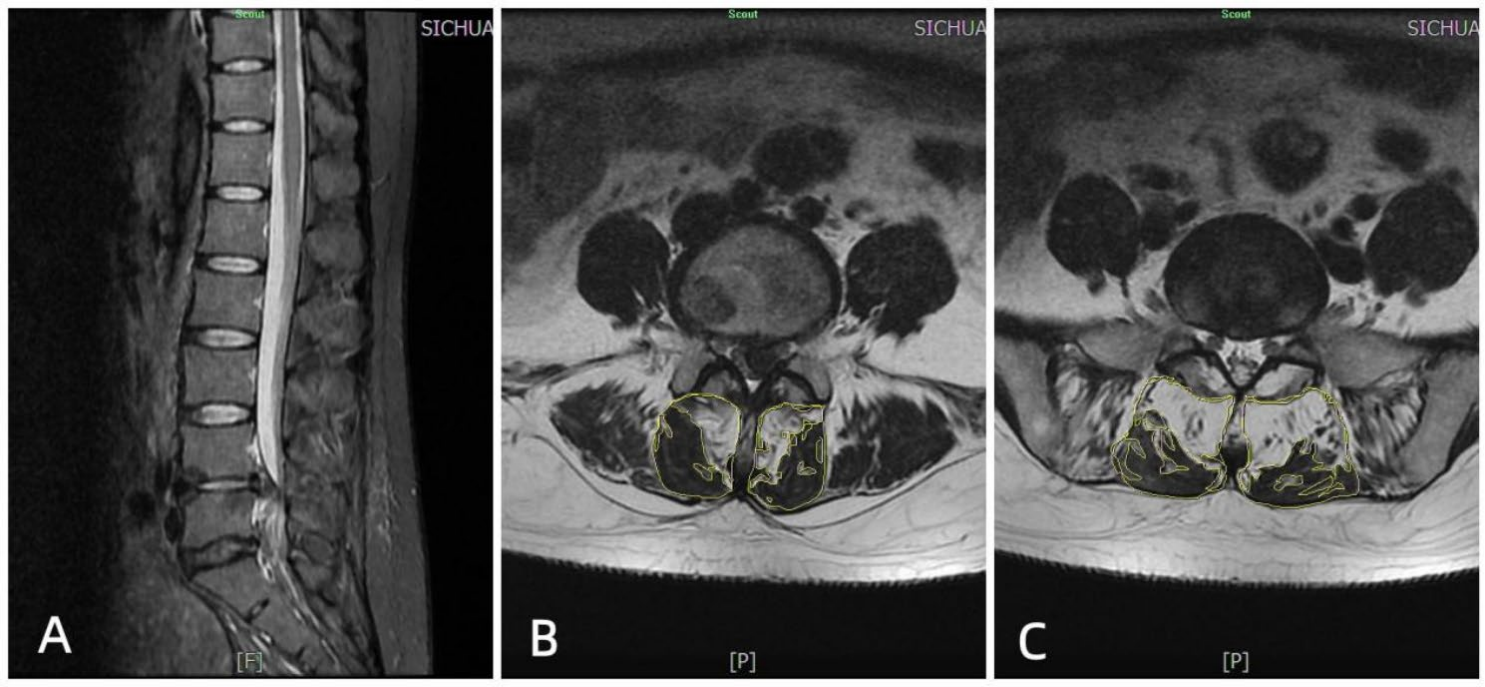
Shows random a L4/5 disc herniation. Figure A shows sagittal MRI. Figure B shows the percentage of fat in the multifidus muscle at the L4/5 level in this patient (39.4% on the affected side and 36.8% on the healthy side), and Figure C shows the percentage of fat in the multifidus muscle at the L5/S1 level in this patient (49.4% on the affected side and 42.6% on the healthy side)
